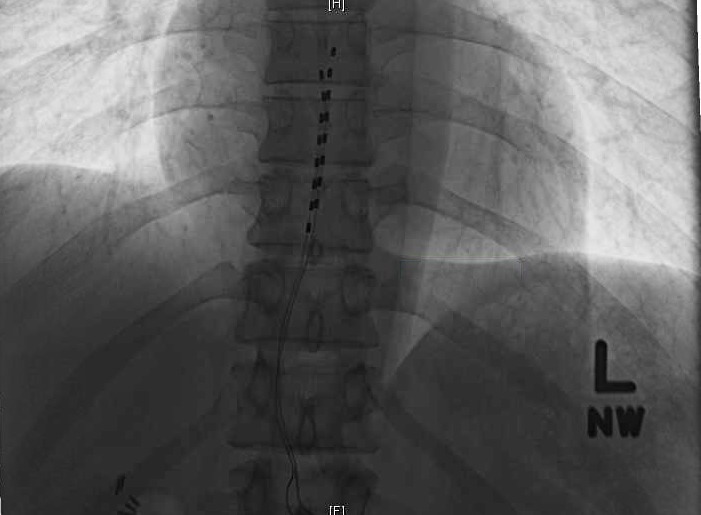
Case 2: Postoperative AP X-ray showing minor lead shift without lateral migration.
